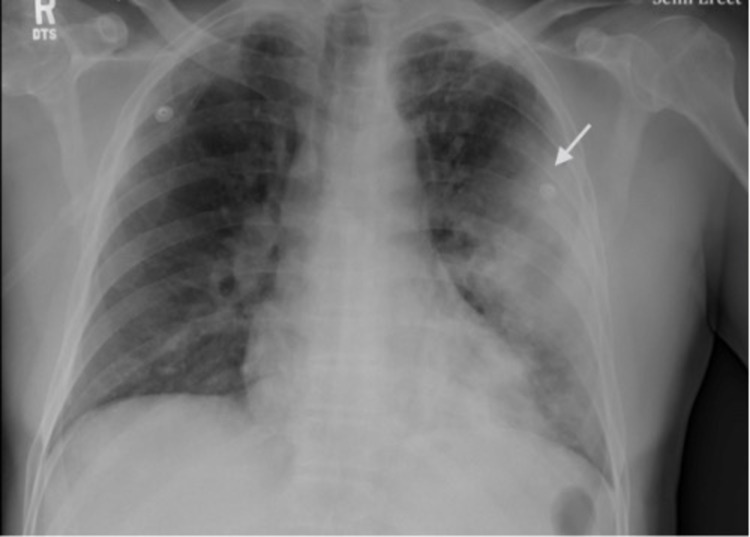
Initial chest x-ray showing large airspace consolidation in the left midlung which has a cavitary appearance. Biapical reticular opacities, left more than right.
